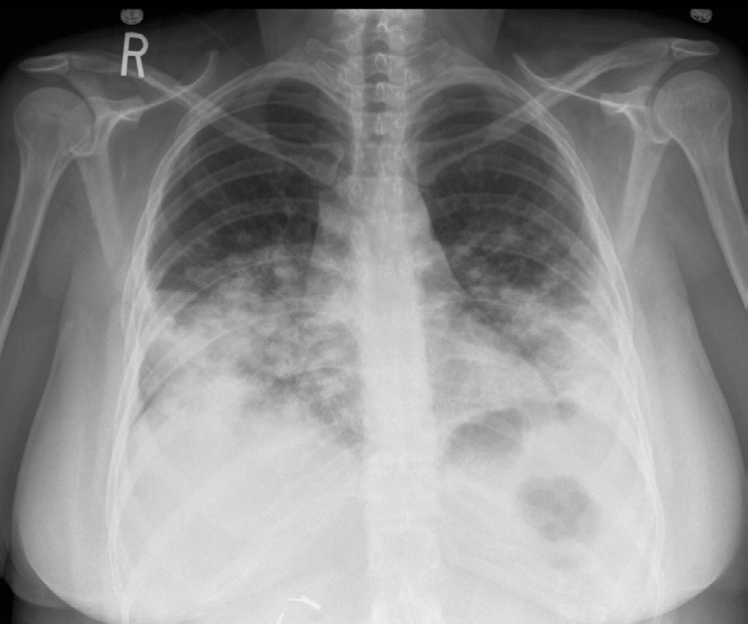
Chest X-ray (PA view) – after 8 days of intravenous antibiotic therapy, showing further worsening of bilateral lower lung disease with nodular pattern – raising suspicion of metastatic disease.
